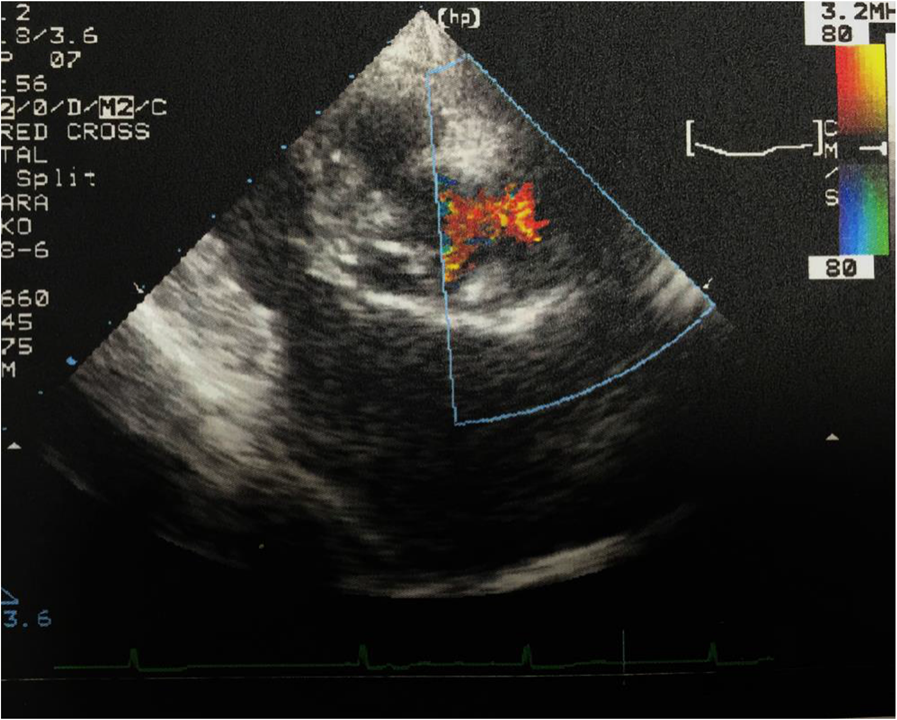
Transthoracic echodardiography. Color-flow doppler shows mosaic pattern in the felt strip on the ascending aorta
